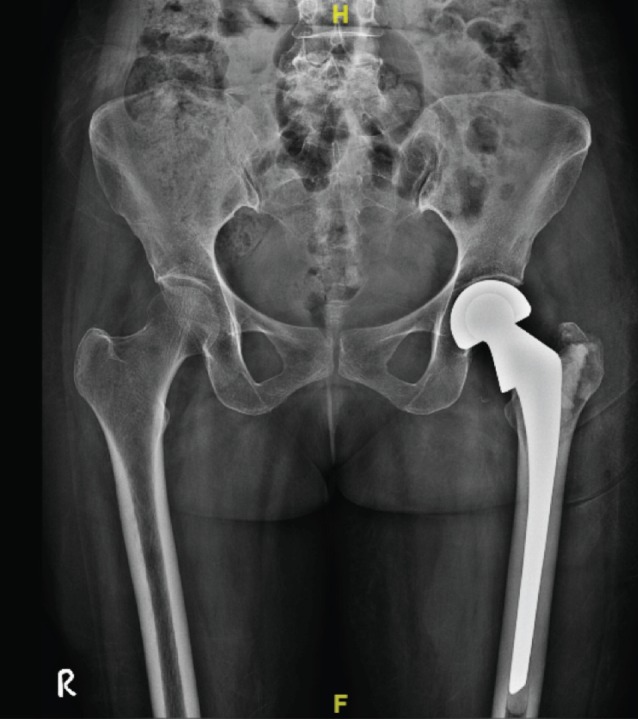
Plain AP radiograph of the pelvis in the immediate postoperative period with bipolar prosthesis in situ.
